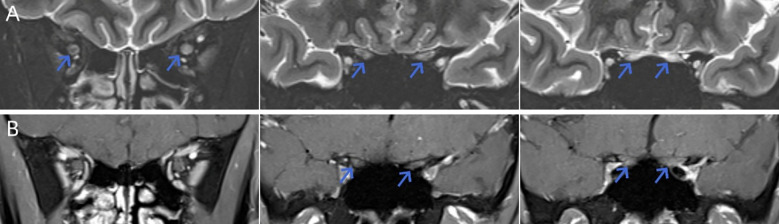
Coronal orbital MRI revealing bilateral optic nerve involvement. (A) Coronal fat-suppressed T2-weighted image demonstrates hyperintensity (blue arrows) involving multiple segments of both optic nerves. (B) Corresponding coronal contrast-enhanced T1-weighted image shows mild linear enhancement (blue arrows) along the affected nerve segments, consistent with active demyelination. MRI, Magnetic Resonance Imaging.
